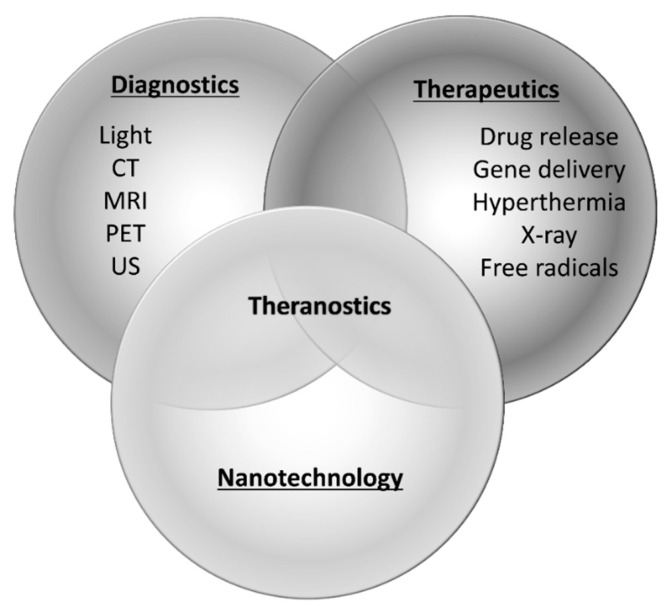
Paradigm of theranostics. CT; computed tomography, MRI; magnetic resonance imaging, PET; positron emission tomography, US; ultrasound.
